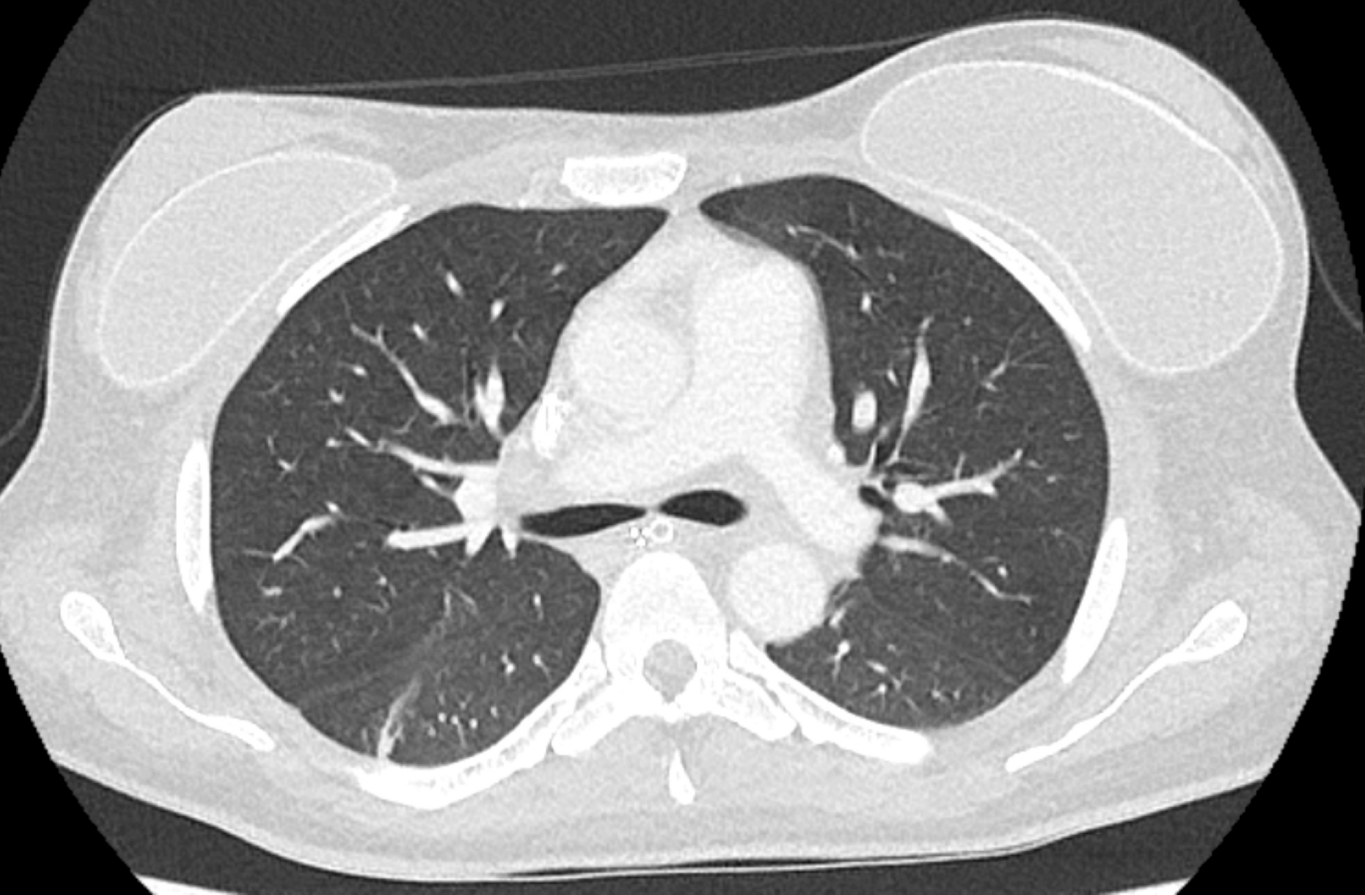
A chest CT scan showed no abnormalities.
